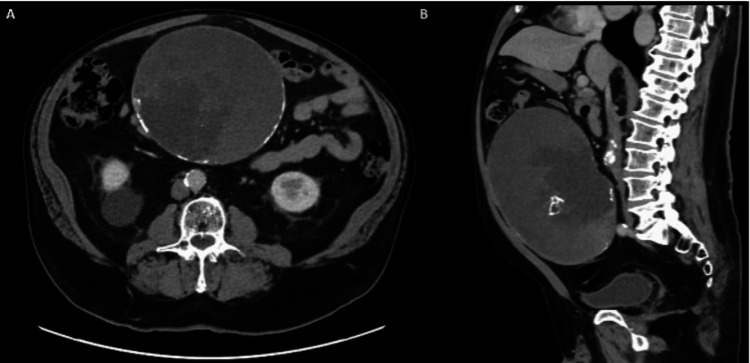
Computed tomography of the abdomen and pelvis with contrast (Case 1). (A) Sagittal view and (B) axial view demonstrating a 16.6 x 18 x 20.9 cm, partly calcified, circumscribed heterogeneous intraperitoneal mass later found to be an appendiceal mucinous neoplasm.
